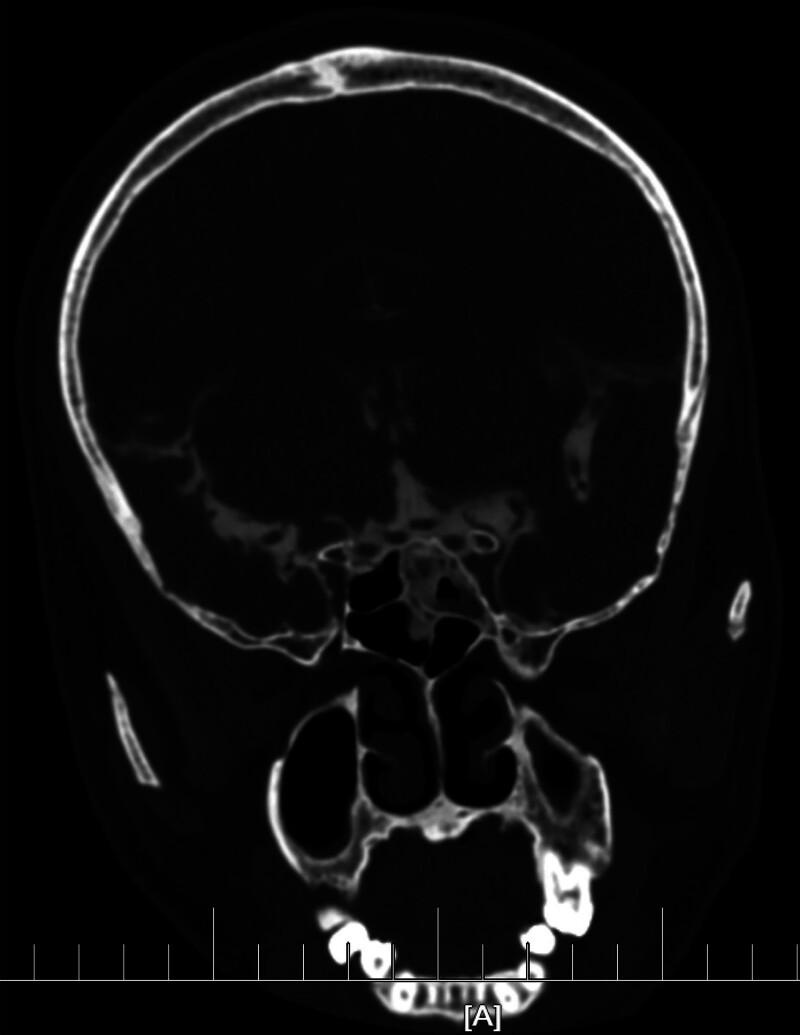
On the CT image of the patient on September 20th, ventricular angiography showed that the ventricular system, sulcus and cistern were filled with contrast agent, and the bone on the top wall of the right sphenoid sinus was discontinuous, with local bone defect shadow, and the contrast agent shadow was connected with the high density shadow of the right sphenoid sinus. CT = computed tomography.
